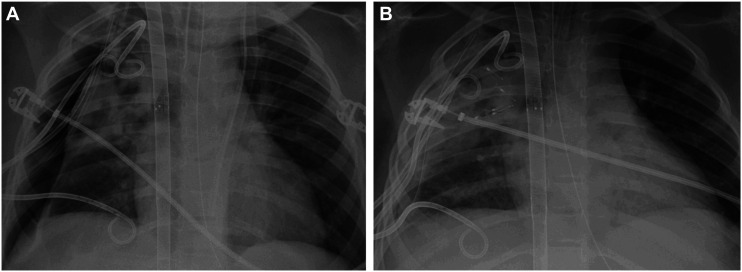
(a) Chest X-ray demonstrating persistent pneumothorax in a mechanically ventilated patient on venovenous ECMO with 3 right-sided chest tubes. (b) Same patient after placement of endobronchial valves.
